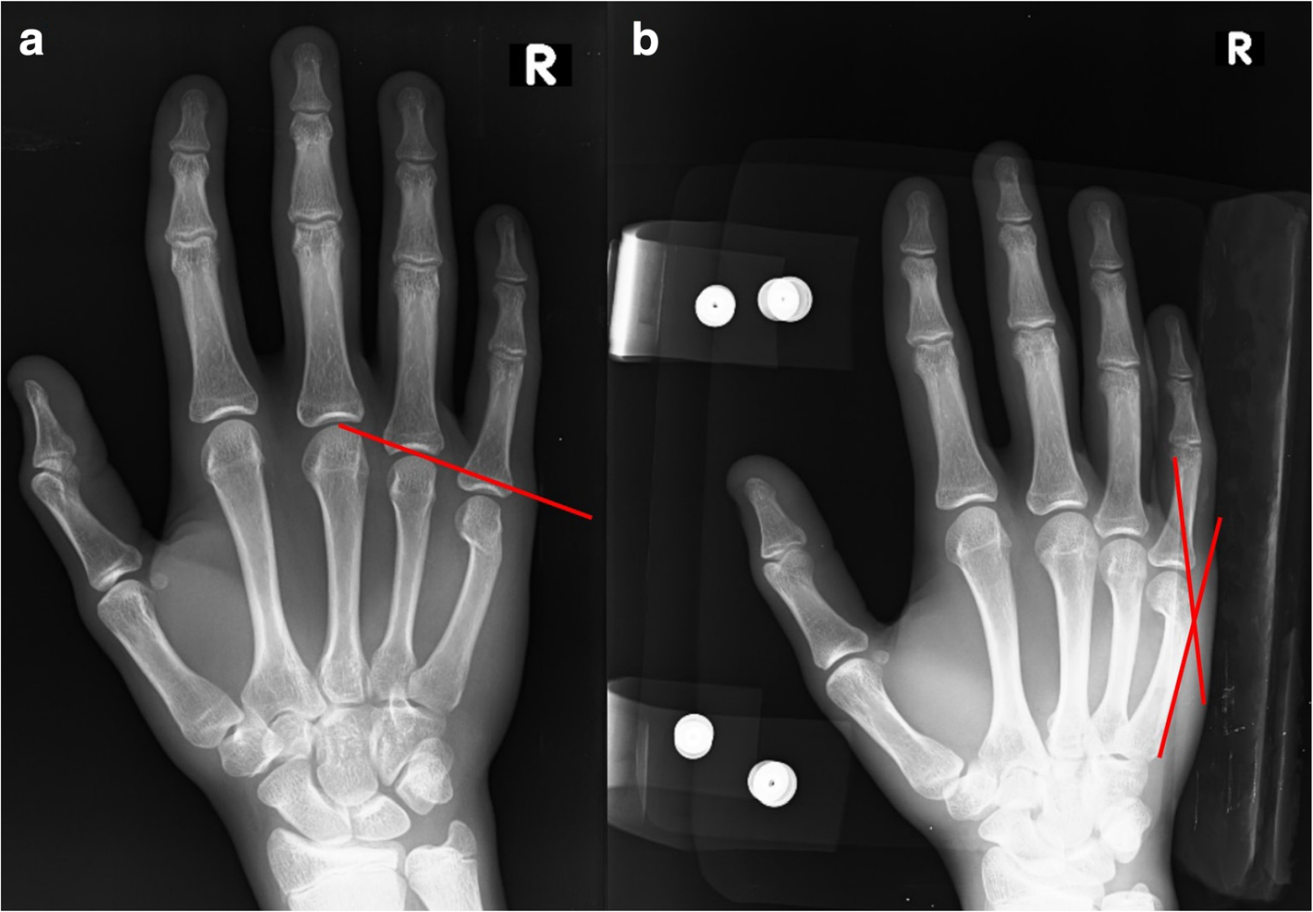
a Method of Shortening Stipulated (SH – Stip), which is used for the measurement of shortening on AP radiography. A line was drawn through the most distal point of the heads of the neighboring 3th and 4th metacarpals. The shortening was defined as the distance from this line to the most distal point of the fractured fifth metacarpal [16]. b Method of Dorsal Cortex – oblique (DC – 30), which is used for the measurement of angulation at a 30° oblique view. The measurement lines were drawn at the most dorsal part of the metacarpal cortices [16]
